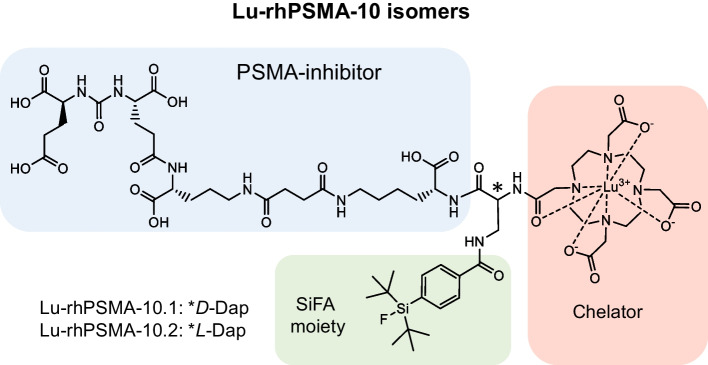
Chemical structure of the Lu-rhPSMA-10 isomers, differing in the stereoconfiguration of diaminopropionic acid (Dap) branching unit. *D*-Dap: rhPSMA-10.1 and *L*-Dap: rhPSMA-10.2. The PSMA inhibitor displays two moieties for radiolabeling: A silicon–fluoride acceptor (SiFA, colored in green), allowing ^18^F-labeling for PET, and a chelator (colored in orange) for complexation of radiometals
